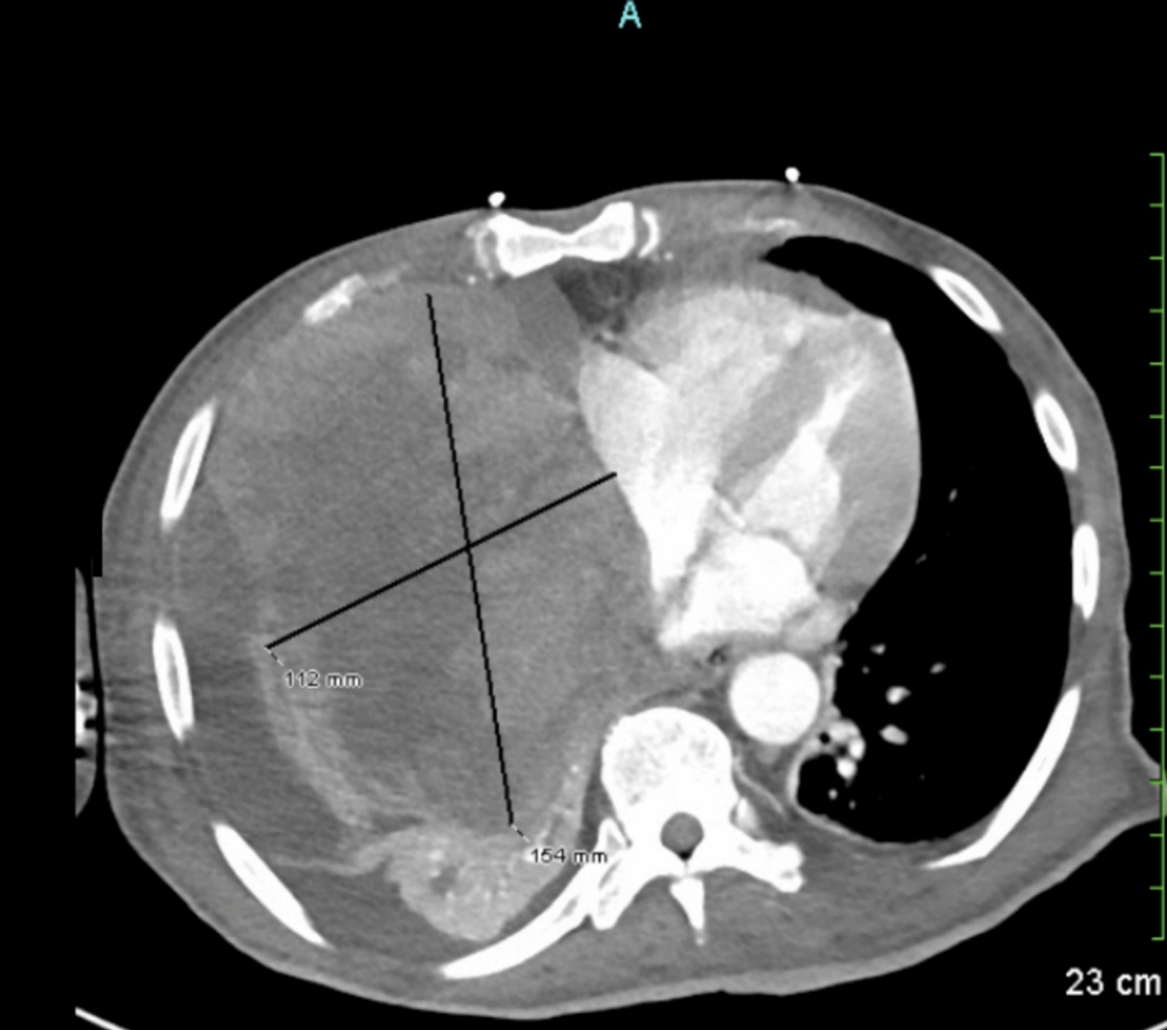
CT scan of the chest showing a large heterogeneous soft tissue tumor within the right thoracic cavity, invading the mediastinum and measuring 11 × 15 × 16 cm. Associated findings include a moderate right malignant pleural effusion, near-total collapse of the right lung, compression and collapse of the right heart chambers, and numerous pulmonary metastases. Numerous osseous metastases are also noted in the cervical spine.
